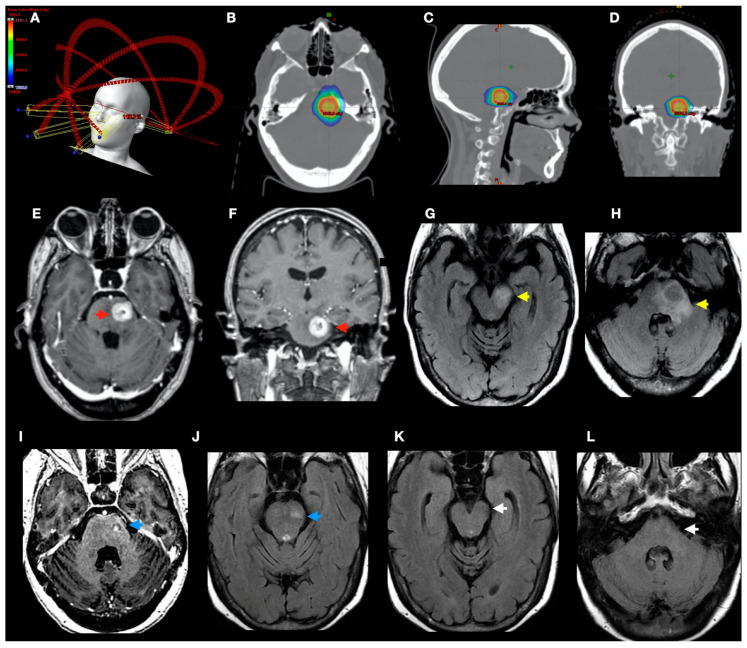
Treatment planning with VMAT-fSRS and local response. (A) Planning example of a patient with one brain metastasis in the pons, treated with fSRS 5 × 6 Gy. Images (B–D) represent axial, sagittal, and coronal plane rotations, respectively. Red PTV outline, green 25 Gy isodose, light blue 20 Gy isodose and dark blue 15 Gy. Pre-treatment MRI (E–H): On post-contrast T1WI, a well-circumscribed lesion (1.7 × 1.7 cm) in the left hemipons shows peripheral ring enhancement consistent with a necrotic metastasis (red arrows, (E,F)). FLAIR images show extensive vasogenic oedema extending along the adjacent cerebellar peduncle and into the ipsilateral pons and midbrain (yellow arrows, (G,H)). Post-treatment MRI (3 months later, (I–L)): On post-contrast T1WI, the left hemipontine lesion decreased significantly with heterogeneous nodular enhancement, and it remained hyperintense on T2/FLAIR (blue arrows, (I,J), respectively). The vasogenic/perilesional oedema resolved across all previously involved regions (white arrows, (K,L)).
